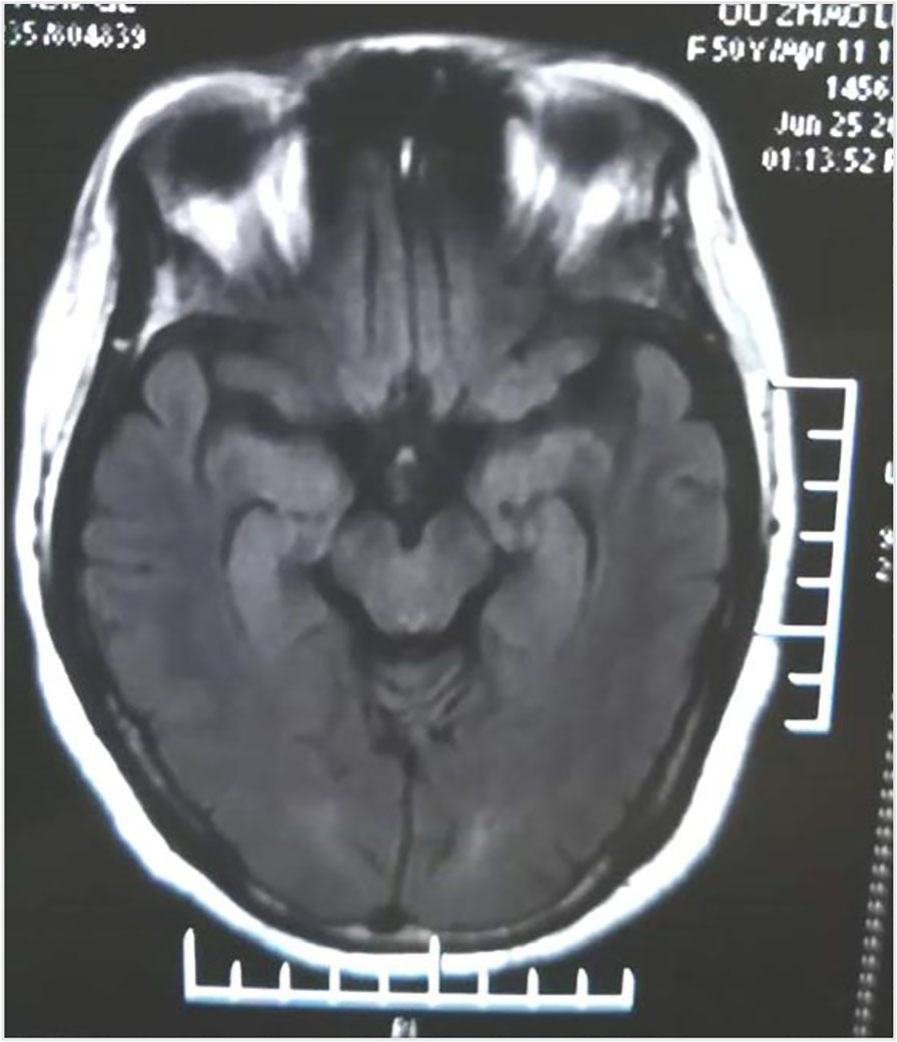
Brain MRI performed on June 25, 2018 identified high-intensity signals on fluid-attenuated inversion recovery in both the medial temporal lobe and hippocampus
